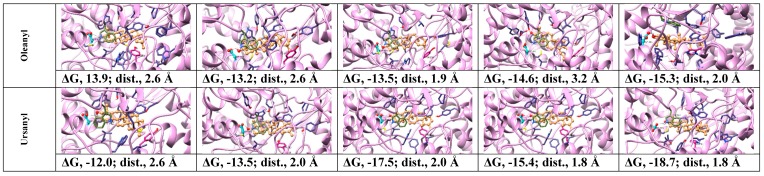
Poses, ligand to protein distances and affinities of oxidosqualene cyclases (OSCs) docked with the intermediate cations from fruit models and human X-ray structure. ΔG, Gibbs free-energy of binding (kcal mol^−1^); dist, ligand-OH.…OOC-Asp-protein distances, ligand, brown; catalytic Asp, cyan; Trp docking reference, green; Tyr/His: magenta. Residues surrounding the active site, dark blue; oxygen atoms, red; nitrogen atoms, light blue and hydrogens, white.
